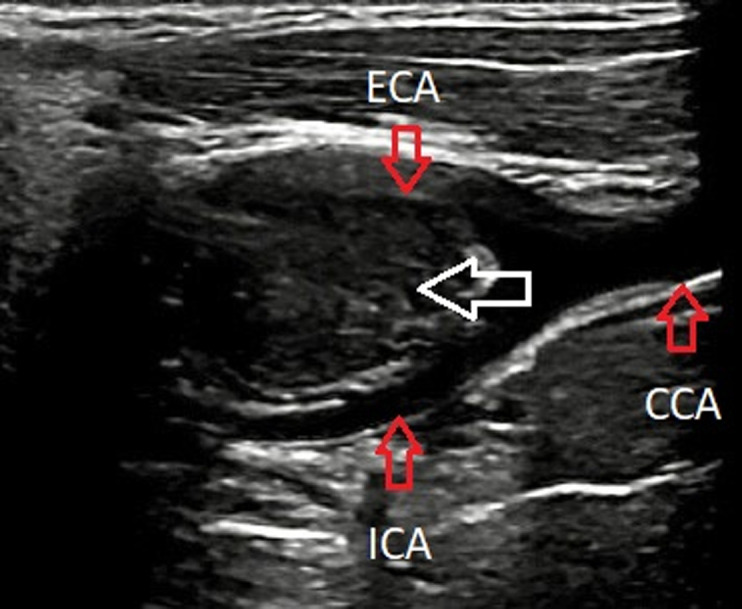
a well-defined homogenously isoechoic lesion above the bifurcation of the right common carotid artery on B mode ultrasonography
